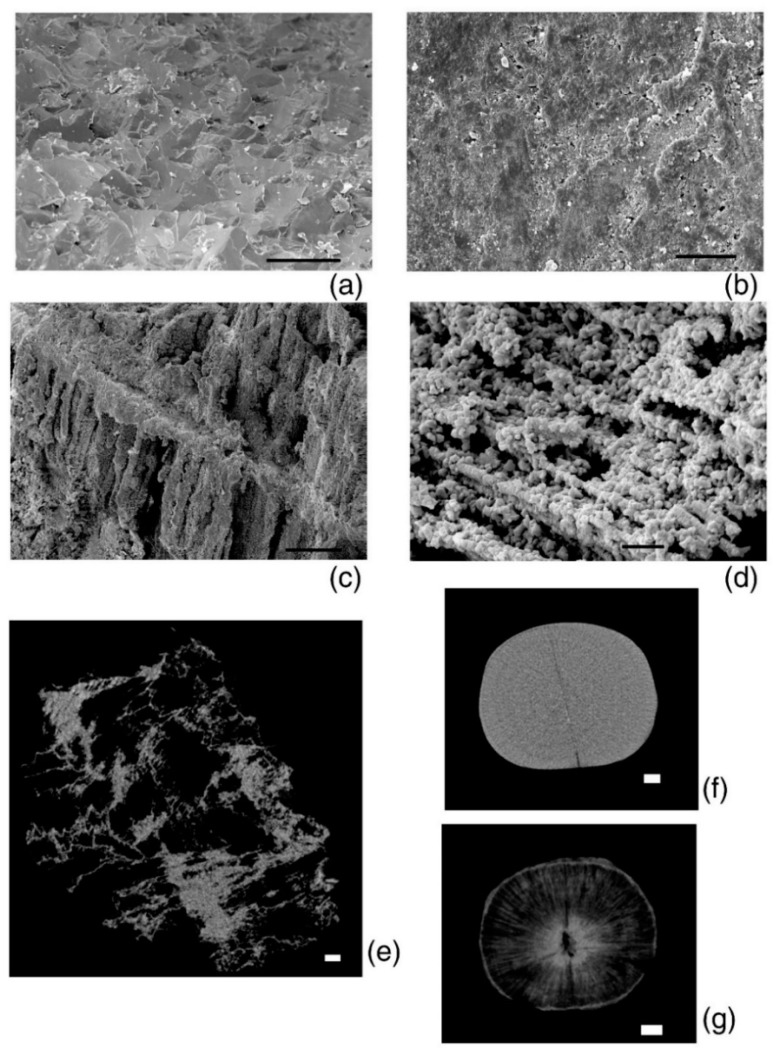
Surface and internal microstructure of belemnite rosters (Upper Jurassic, Upper Volgian; Moscow, Kuntsevo–Filyevsky Park) substituted and unsubstituted with iron minerals: (a) transverse split of belemnite rostra not substituted with iron minerals, scale is 100 µm; (b) surface of belemnite rostra not substituted with iron minerals, scale is 30 µm; (c,d) microstructure of the rostrum substituted with iron minerals, scales are 100 and 10 µm, respectively; (e) virtual section of a growth fragment substituted with iron minerals, X-ray microtomography, almost all calcite structures are destroyed inside, scale is 100 µm; (f) transverse virtual section of a belemnite roster not substituted with iron minerals, X-ray microtomography, scale is 1 mm; (g) transverse virtual section of the belemnite rostrum substituted with iron minerals, X-ray microtomography, scale is 1 mm.
